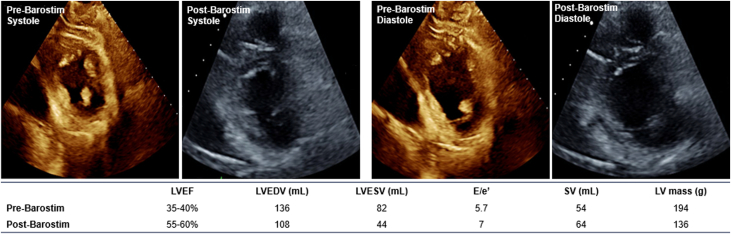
Case 2: Echocardiographic Images of Pre- and Post-Barostim Implant During Systole and Diastole With Associated Clinical Metrics of Interest
